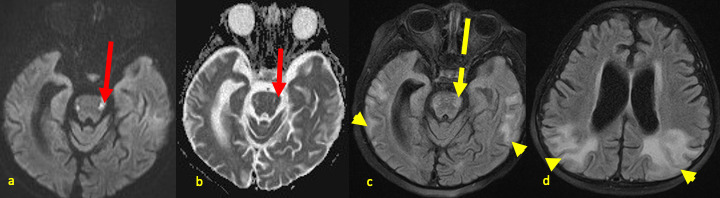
Now 35-month-old male with rAHNE. Follow-up brain MRI obtained 18 weeks after the last acute episode displayed in Figure 3, axial images demonstrating evolving sequelae of hemorrhagic necrotizing encephalopathy: DWI (a) & ADC (b) showing bilateral signal abnormalities in lateral pons (red arrows); FLAIR images (c, d) showing new cortical/subcortical hyperintense signal abnormality in the temporal and parietal lobes (arrow heads), with decreasing pontine signal abnormality (broken arrows). Note the worsening diffuse parenchymal volume loss with ex-vacuo dilatation of lateral ventricles and widening of sulci. ADC, apparent diffusion coefficient; DWI, diffusion-weighted image; FLAIR, fluid attenuation inversion recovery; rAHNE, recurrent acute hemorrhagic necrotizing encephalopathy.
